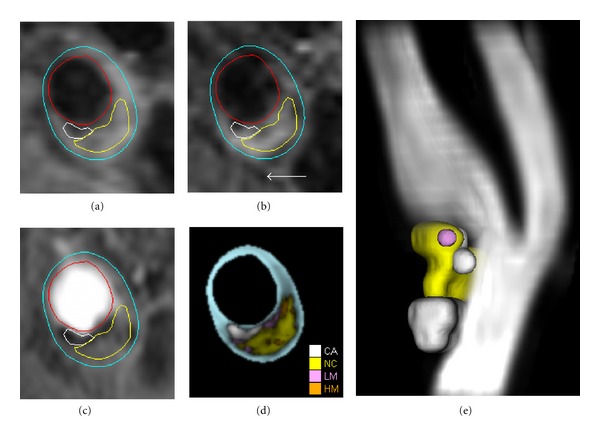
Example of multicontrast MRI characterization of carotid plaque composition showing (a) T1-weighted, (b) T2-weighted, and (c) time-of-flight images of the carotid artery. Automated segmentation with MEPPS [78] yields (d) a color-coded map indicating locations of calcification (CA), necrotic core (NC), loose matrix (LM), and hemorrhage (HM). Combination of results from cross-sections spanning the length of the plaque produce (e) a 3D rendering of the plaque components relative to the angiographic view of the carotid bifurcation (rendered with MRI-PlaqueView, VPDiagnostics Inc., Seattle, WA).
